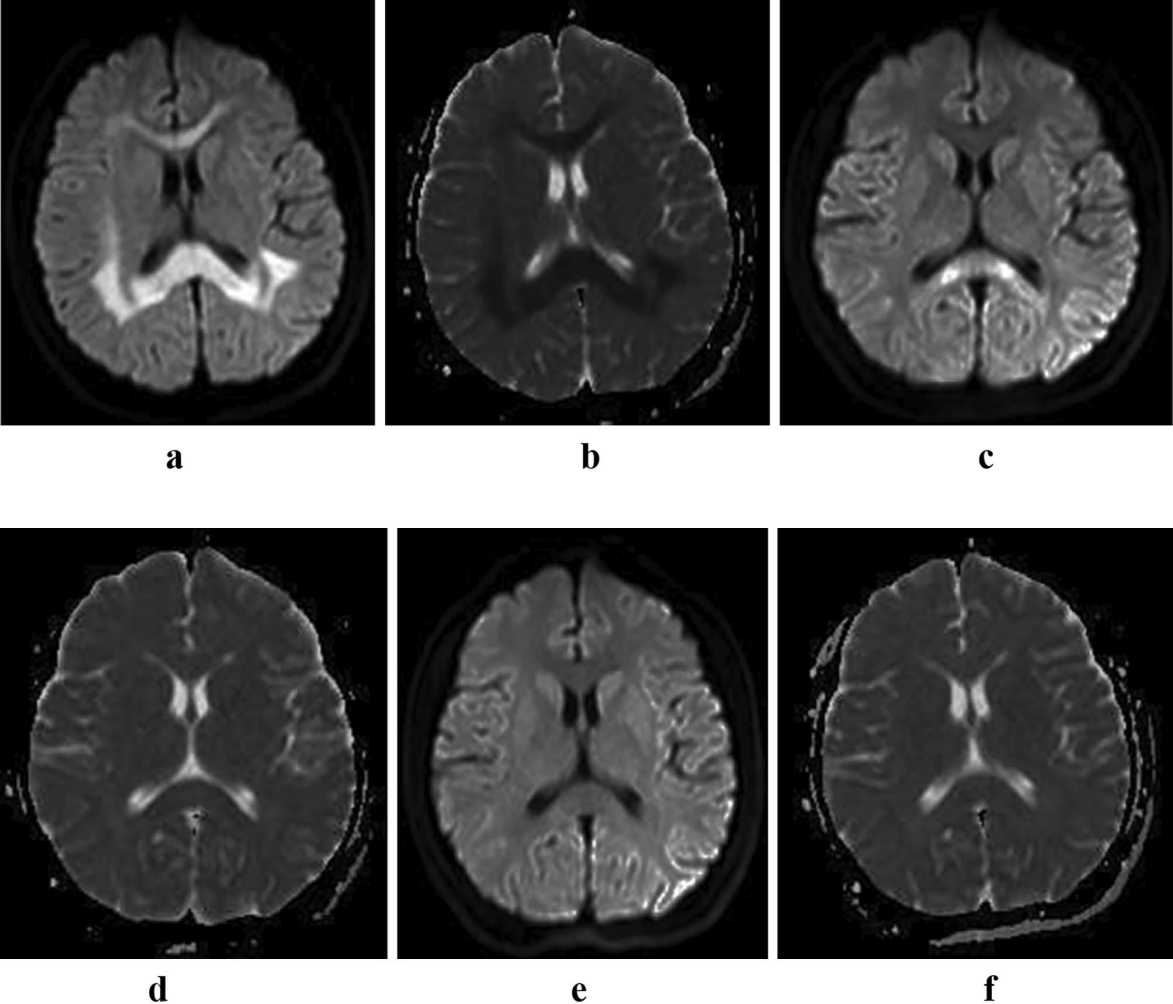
Type A acute MBD. Axial MRI scans showed extensive swelling of the corpus callosum with marked hyperintensity on DWI (a) and hypointensity on the apparent diffusion coefficient (ADC) map (b). Symmetrical restricted diffusion could be also observed in the bilateral hemispheric white matter (a,b). Six days after treatment, most intracerebral hyperintensity on DWI disappeared, except for the splenium (c), but it was unclear on the ADC map (d). Ten days later, the DWI hyperintensity in the splenium disappeared completely. Both DWI (e) and ADC map (f) were displayed normally.
